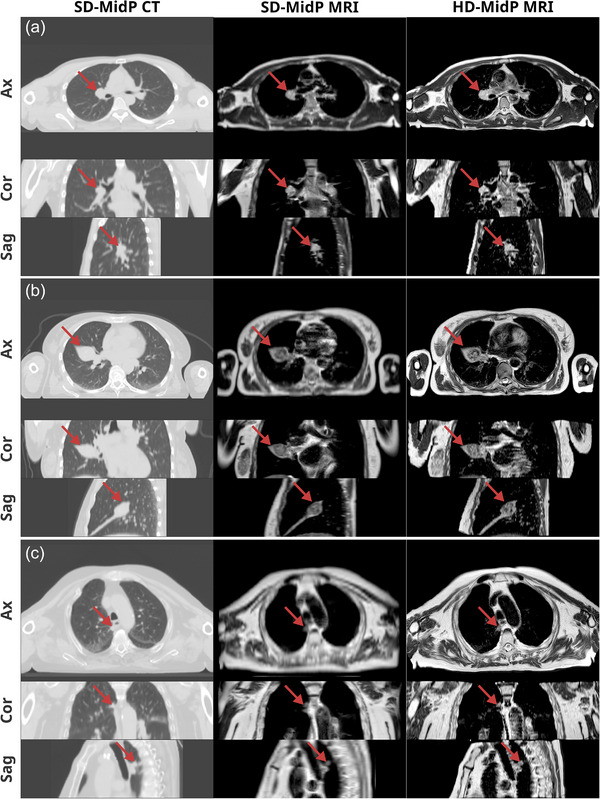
Examples of mid‐position (MidP) images of lung cancer patients for the standard‐definition (SD) and high‐definition (HD) approaches. The axial (Ax), coronal (Cor), and sagittal (Sag) planes intersecting the tumor location are shown. The arrows highlight the tumor location. The SD‐MidP CT is visualized using lung windowing (W:1700, L:−500), whereas MidP MR images have identical windowing (W:1860, L:1070). These examples were derived from 4D‐MRI scans with coronal slice thickness values of 4.0 mm (P2, a), 4.5 mm (P10, b), and 6.0 mm (P12, c).
